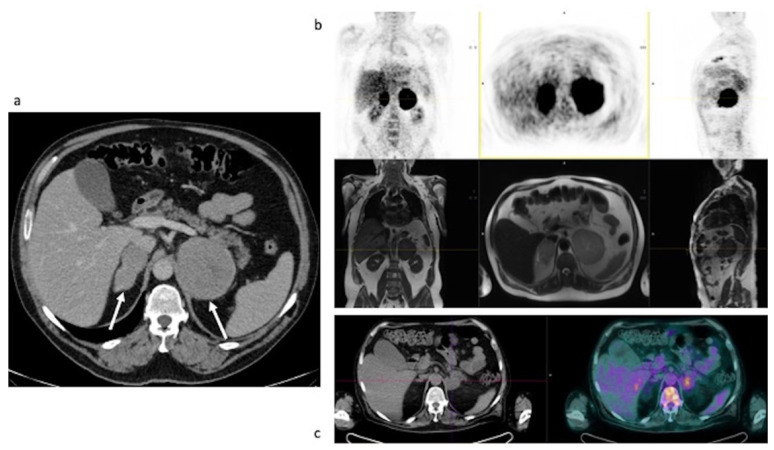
Case #3. Baseline CT (a), baseline FDG PET/MR (b) and post-therapy FDG PET/CT (c). The arrows demonstrated the left and the right adrenal lesions.
